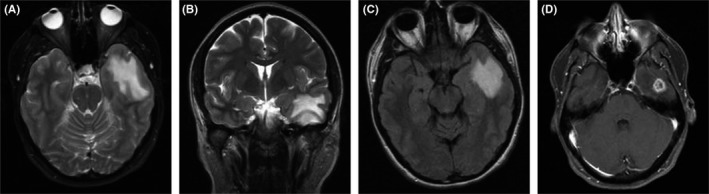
Preoperative MRI. A, Axial and B, coronal T2 MRI demonstrated a hyperintense left temporal lobe lesion. C, Perilesional hyperintensity on FLAIR sequence suggestive of vasogenic edema. D, Postcontrast T1 MRI revealed a solitary enhancing left temporal lobe lesion
